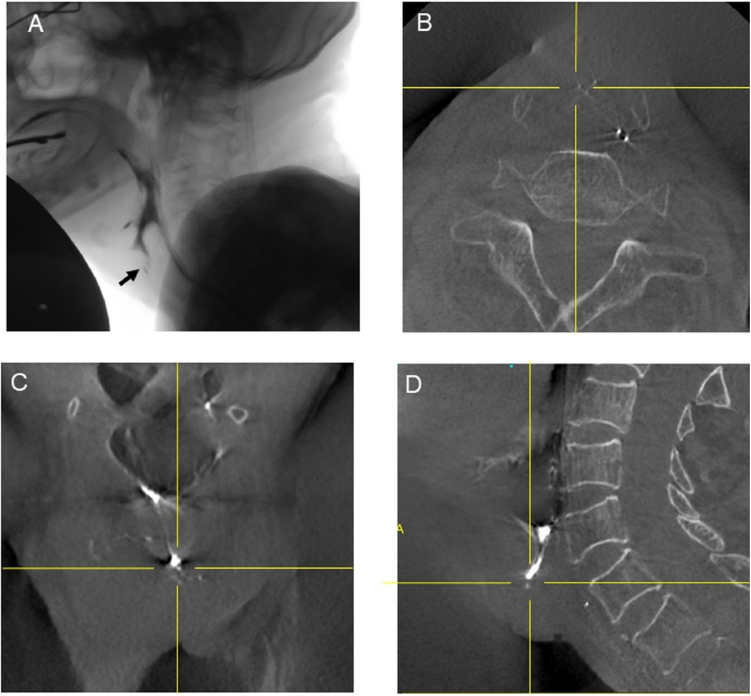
Videofluoroscopy and contrast CBCT findings of Case 4. A, Videofluoroscopy showed a suspicious minor pharyngeal leakage. The black arrow indicates the suspicious leakage. (B–D) The intersection of the vertical and horizontal yellow lines indicates the same location. CBCT detected no leakage.
